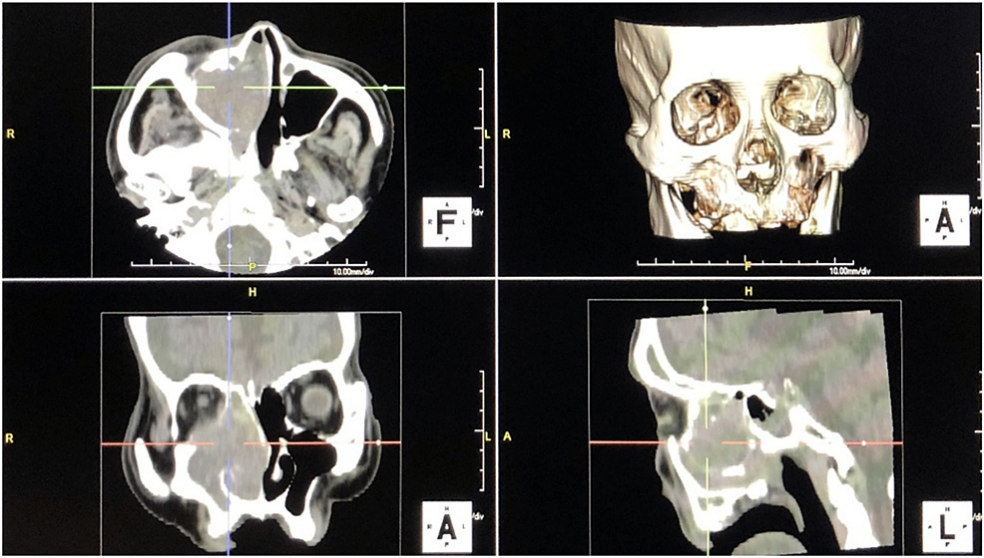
Three-dimensional CT findings of the sinus cavity CT, computed tomography.
